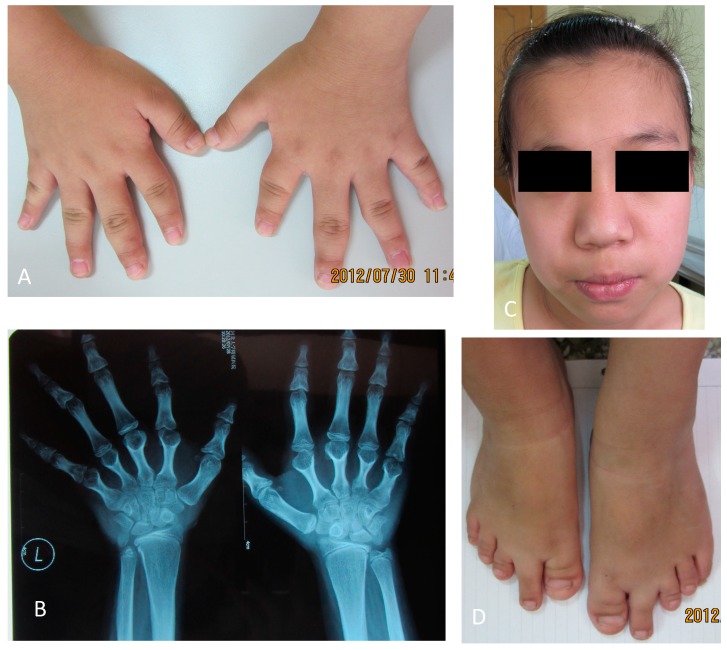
Patient photographs and X-ray images. (A) Small hands; (B) X-ray of the patient’s hands shows shortened metacarpals; (C) Round face and “pug-nose” can be observed in the patient’s photograph; and (D) Photograph of the patient’s feet shows shortened toes and metatarsals.
